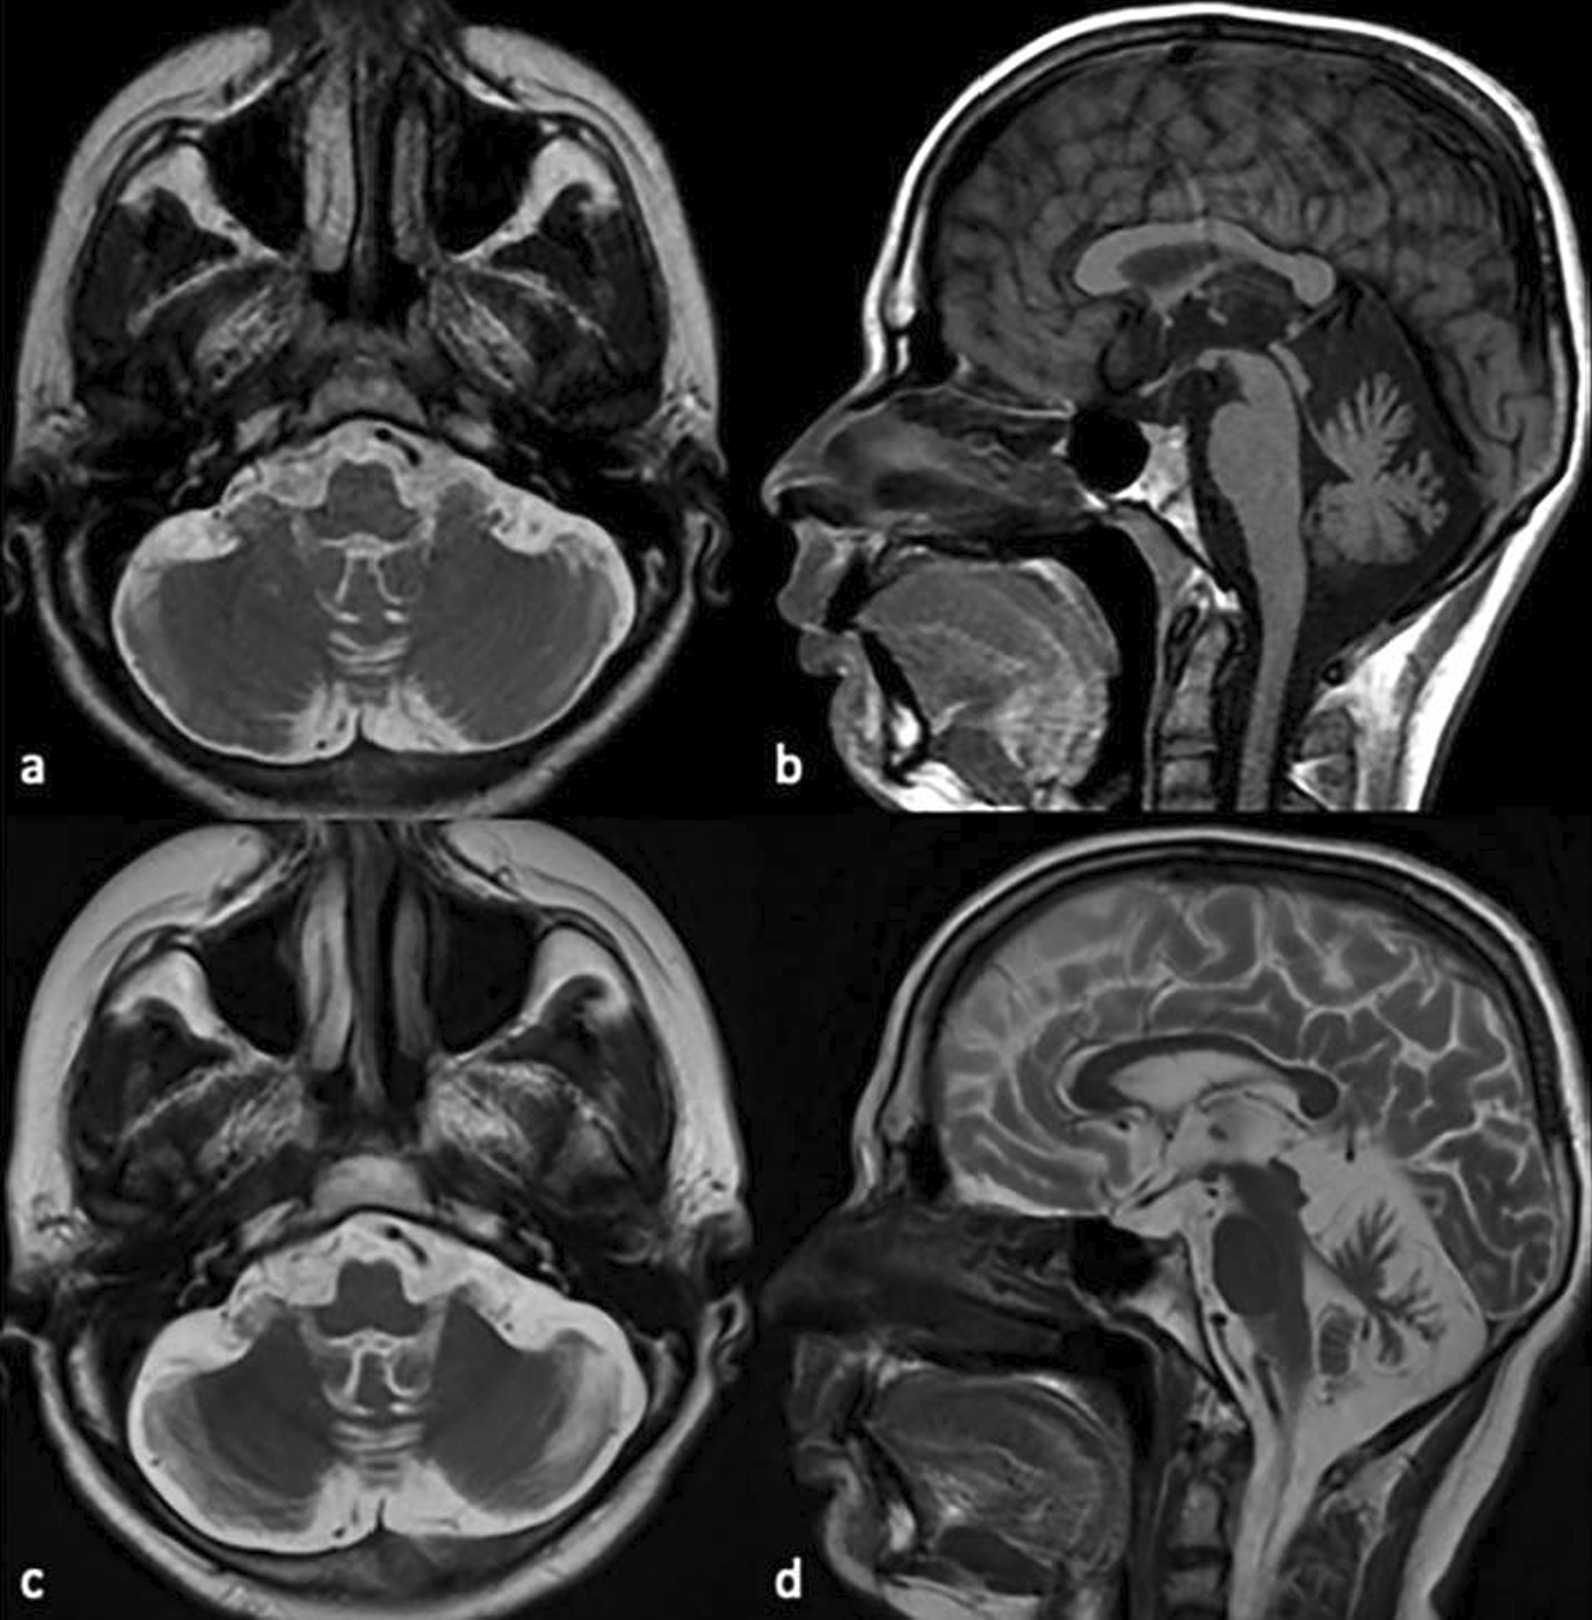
MRI of the case in 2018 and 2022. According to 2018 (a, axial T2 and b, sagittal T1-weighted) MRI in 2022 (c axial, d sagittal T2-weighted) progression is seen in cerebellar, vermian and cerebral cortical atrophy. Mild atrophy in the mesencephalon has not progressed
